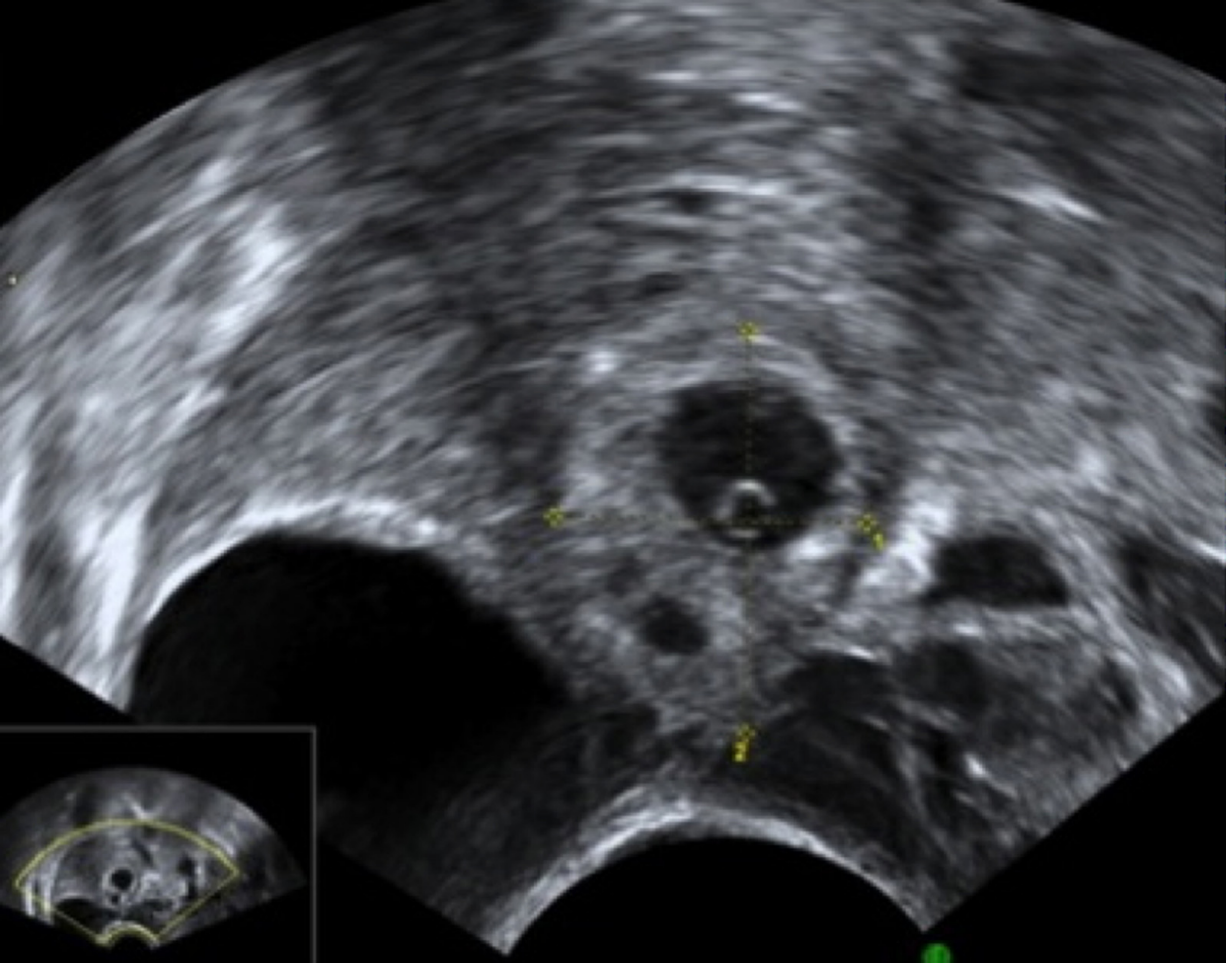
Ultrasound image of a right tubal ectopic pregnancy at 6^+2^ weeks gestation. A gestation sac is seen adjacent to the right ovary.
